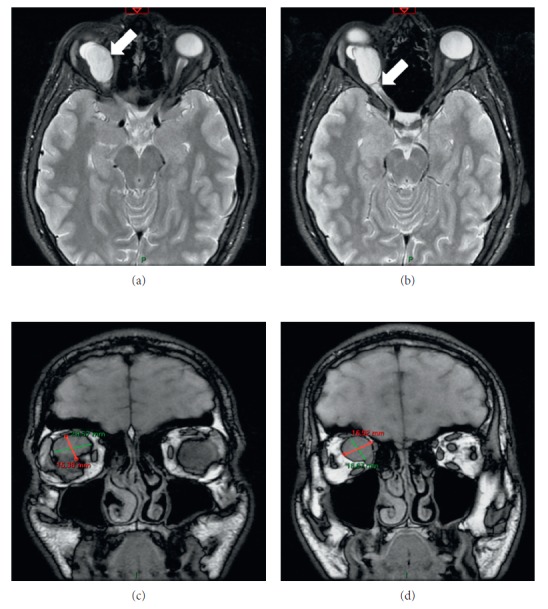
Preoperative MRI scans. (a) A well-defined tumor (white arrow) with vivid signal intensity in the right orbit. (b) The tumor cannot be separated from the optic nerve, followed by the foramen optic (white arrow). (c) The T2-weighted image shows the exact position and size of the tumor. (d) The dorsal edge of the previous shot.
